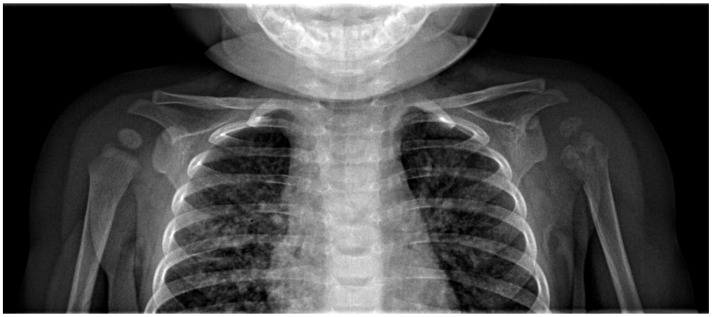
X-ray of the clavicle and left shoulder: demineralization and irregular areas of osteolysis at the diaphyseal-metaphyseal and epiphyseal level, proximal to the left humerus.
